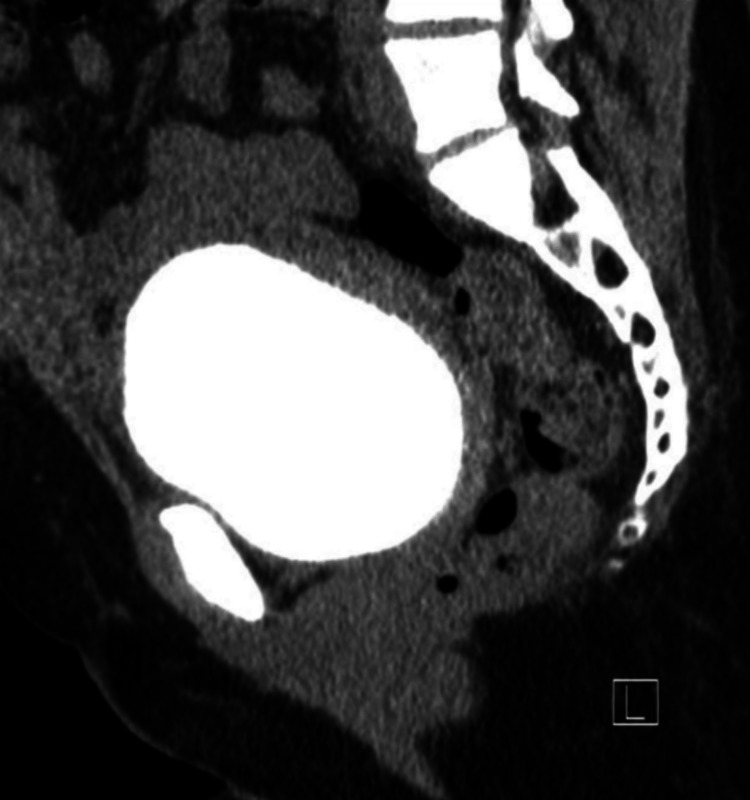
Sagittal view of the repeated CT cystogram after four weeks from the initial study showing a fully opacified, well-rounded urinary bladder with no evidence of contrast leakage and resolution of the anterior pelvic collection.
